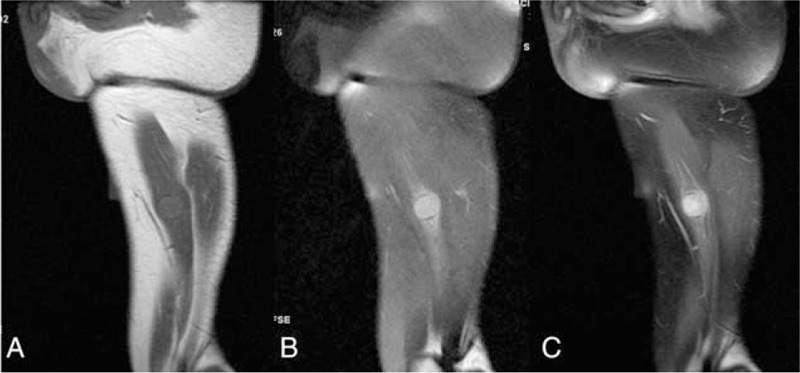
Magnetic resonance imaging (MRI) of the nodule in the right adductor magnus. (A) T1WI showed the homointensity of the nodule in the right adductor magnus. (B) T2WI showed the moderately high intensity. (C) Contrasted-MRI showed a homogeneous enhancement.
